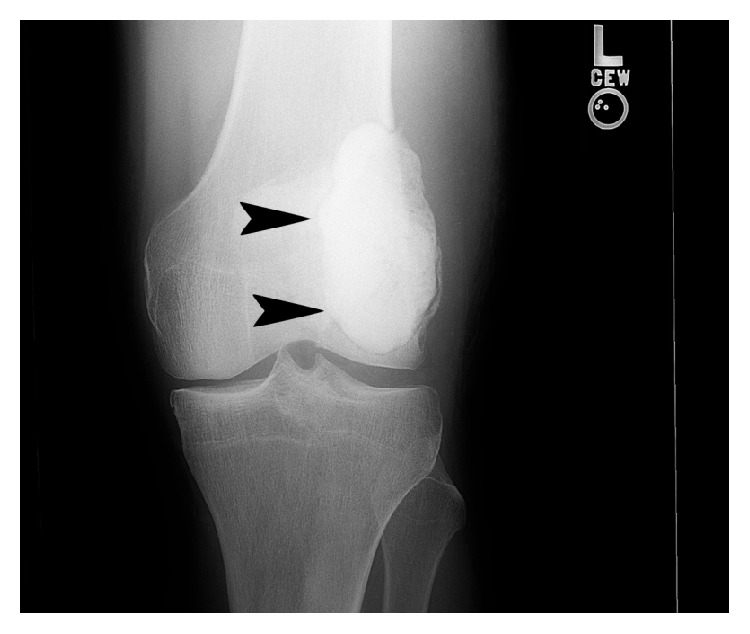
Frontal left knee radiograph (August 2007) demonstrates postsurgical curettage and packing with radiopaque polymethylmethacrylate (PMMA) of a well-defined, solitary, mixed lytic, and sclerotic lesion with a narrow zone of transition (arrows), located within the distal femoral metaphysis.
